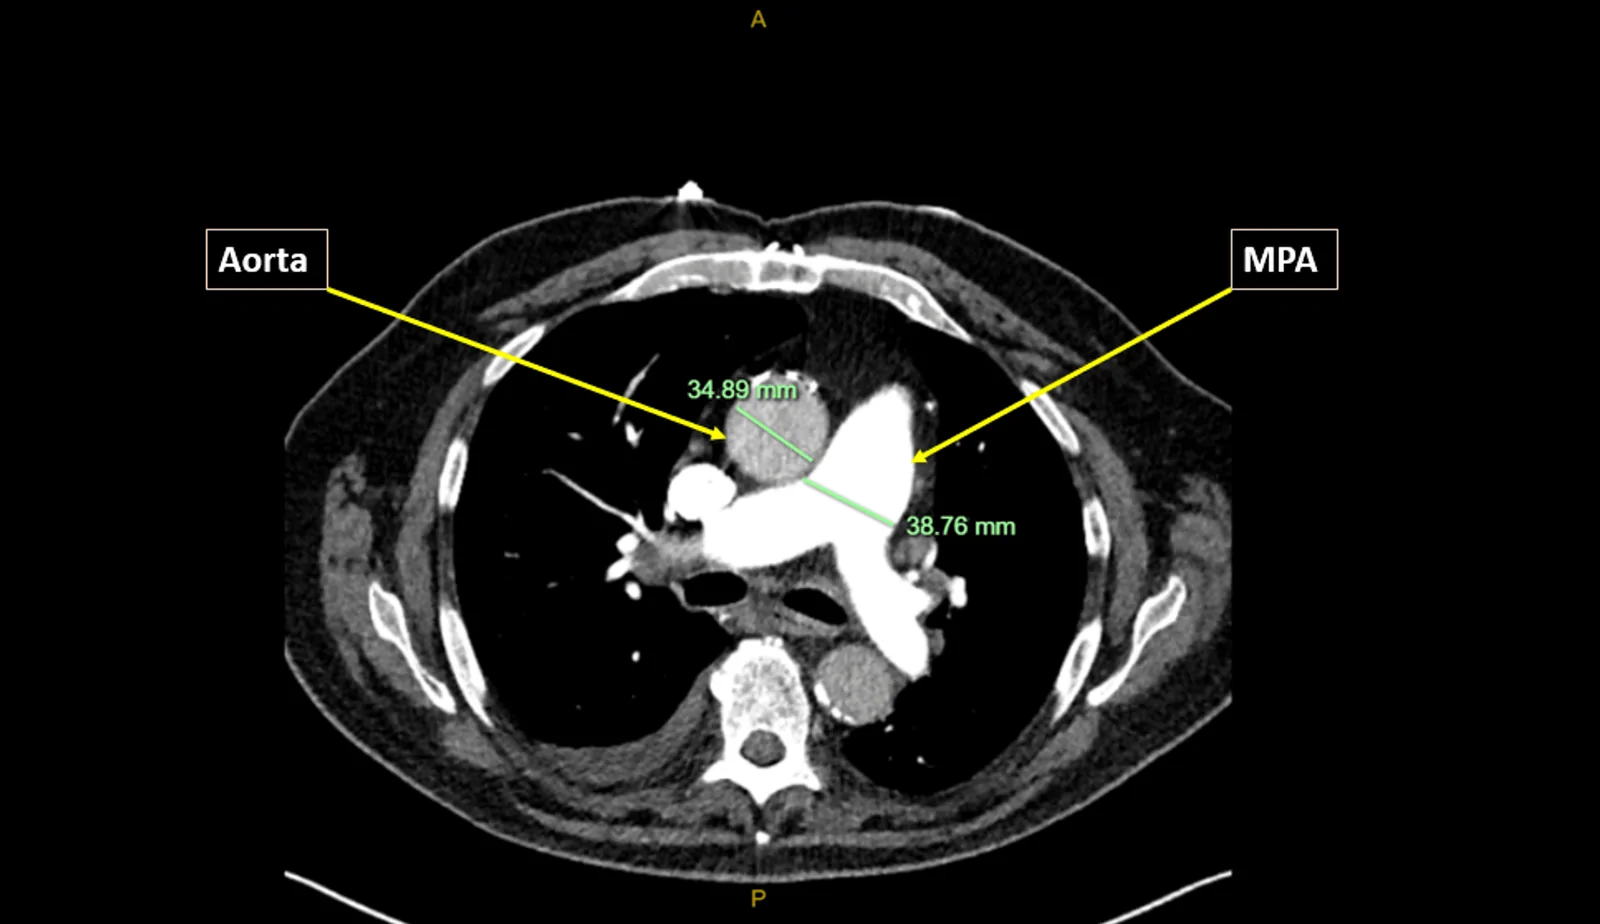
Contrast-enhanced CT chest demonstrating main pulmonary artery dilatation Axial contrast-enhanced CT pulmonary angiography demonstrating main pulmonary artery (MPA) dilatation measuring 38.76 mm, exceeding the diameter of the ascending aorta (Ao; 34.89 mm), consistent with pulmonary hypertension.
